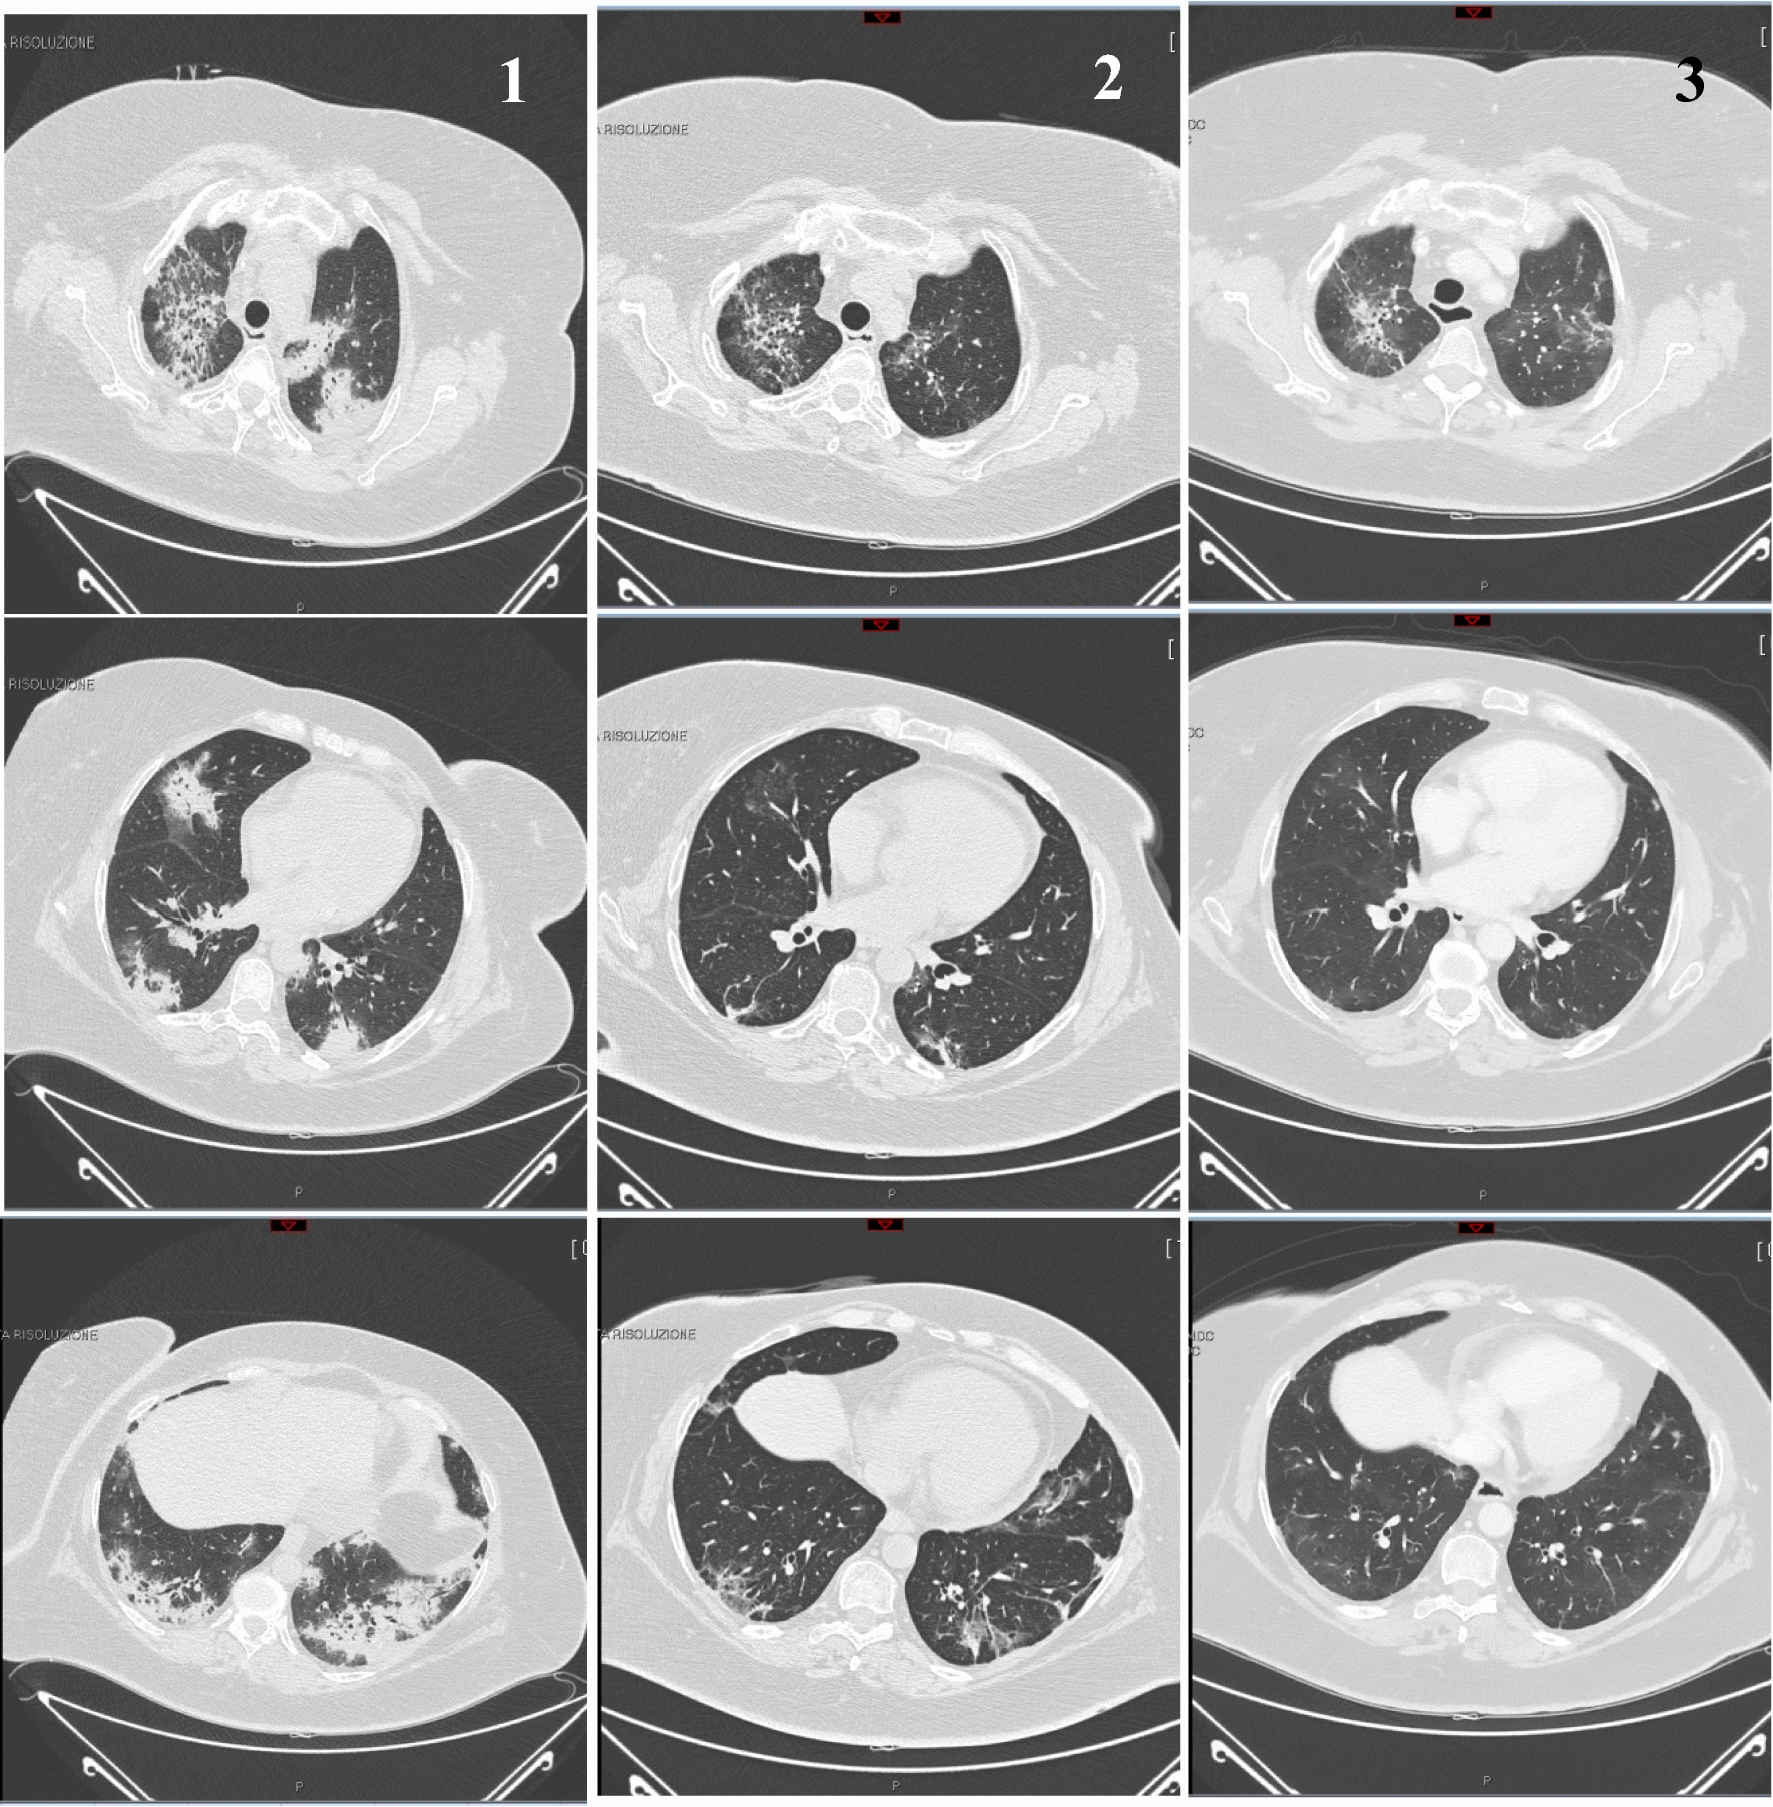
Radiological and cytological features in a representative patient. A. Chest computed tomography at different timepoints. 1. CT images at admission (at 22 months after starting pembrolizumab), showing a COP pattern with multiple pseudo-nodular parenchymal consolidations with irregular and shaded margins, spread bilaterally but more extended to the lower lobes. Ground glass areas were observed in the upper right lobe. 2. CT image at 1 month after the onset of CIP and after i.v. methylprednisolone, showing significant resolution of the bilateral consolidations with minimal resolution of ground-glass opacities. 3. CT image at 4 months after discharge showing further improvement in radiological alterations
